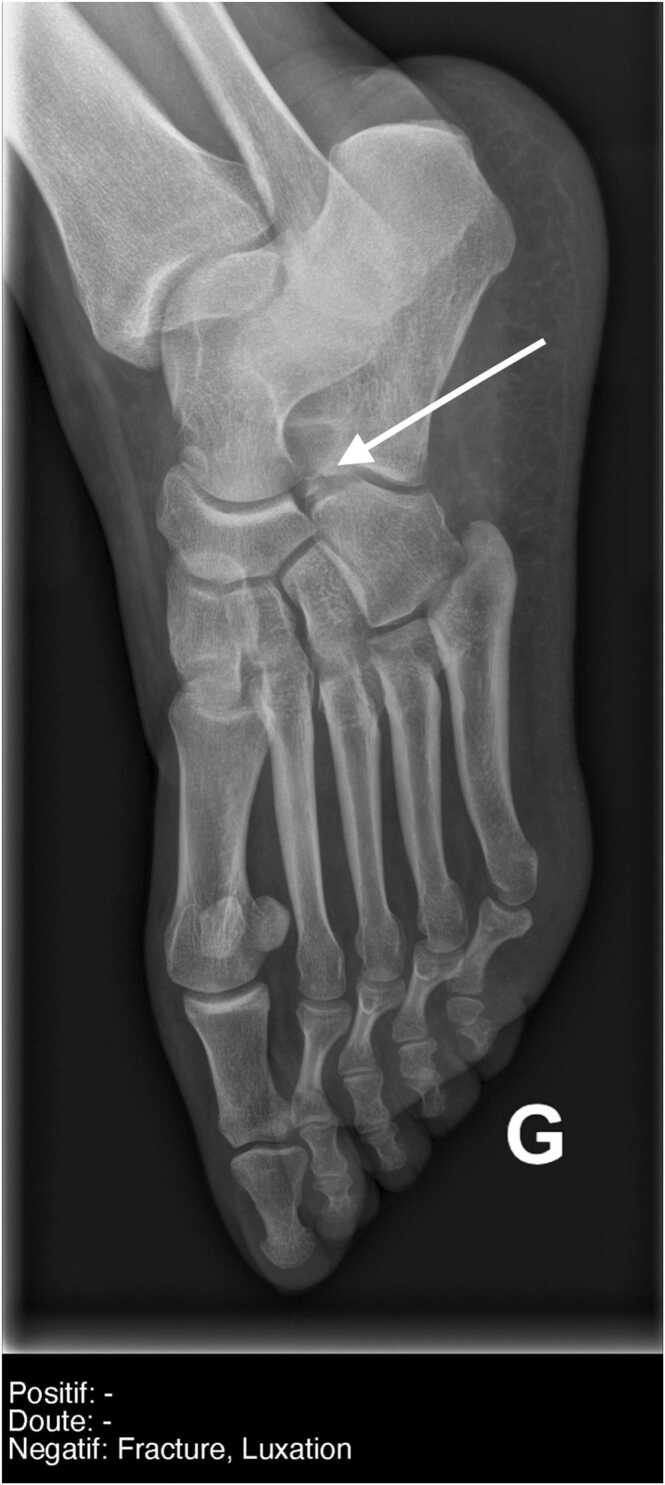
Example of an MSK discordant case missed by AI. 3/4 view of a foot radiograph in an adult patient, reported as normal by the ED and the AI. The radiologist reported an avulsion fracture of the calcaneal rostrum only visible on the 3⁄4 view, related to a Chopart joint sprain (white arrow). French translation: doute = doubt; luxation = dislocation; negatif = negative; positif = positive.
